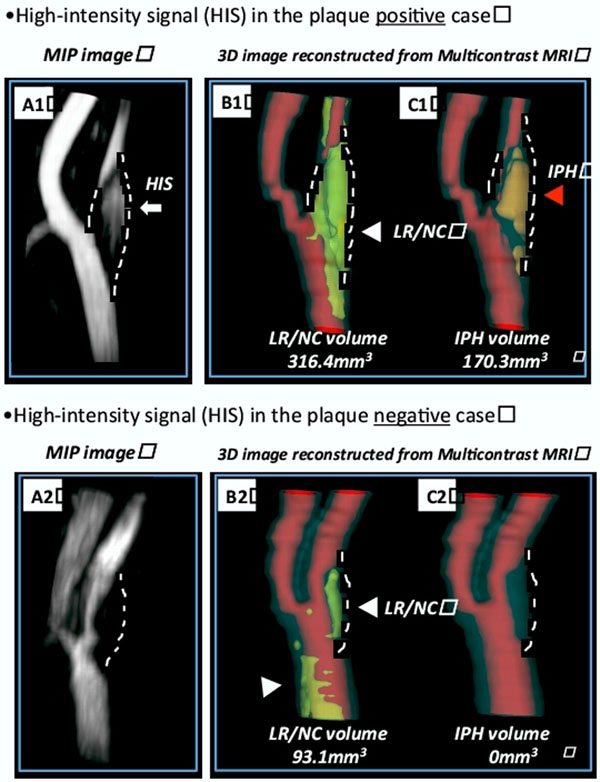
Representative images of high-intensity signal (HIS) positive plaque (A1) and negative plaque (A2). HIS positive plaque is identified as high signal in the vessel wall without connection to the lumen (White arrow). HIS negative plaque has no HIS but shows luminal stenosis. Lipid rich necrotic core (LRNC) (B1&2; ehite arrowhead) and intraplaque haemorrhage (IPH) (C1; Red arrowhead) were displayed using three dimensional reconstruction images of multicontrast MRI. Volume of LRNC and IPH are calculated from cross-sectional areas length of plaque using the computer-aided system for cardiovascular disease evaluation (CASCADE), a specialized software suite for plaque analysis.
